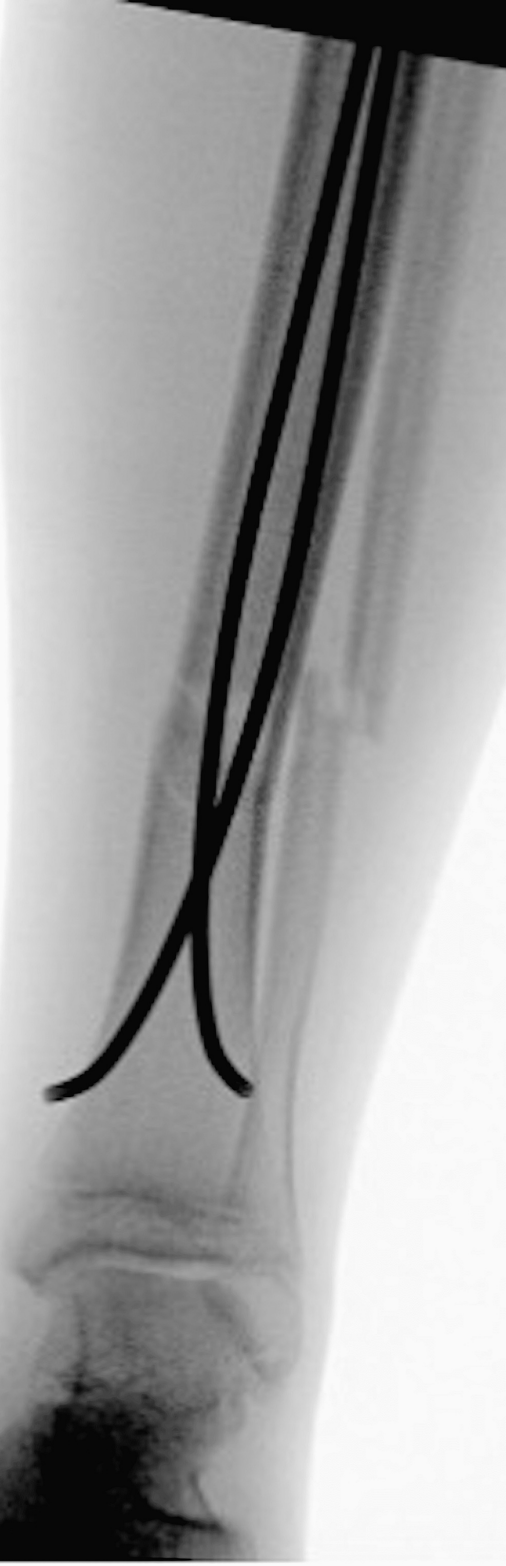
Anterior-posterior tibia-fibular intra-operative radiographs of irrigation and debridement with concomitant flexible nailing of the tibial shaft.
